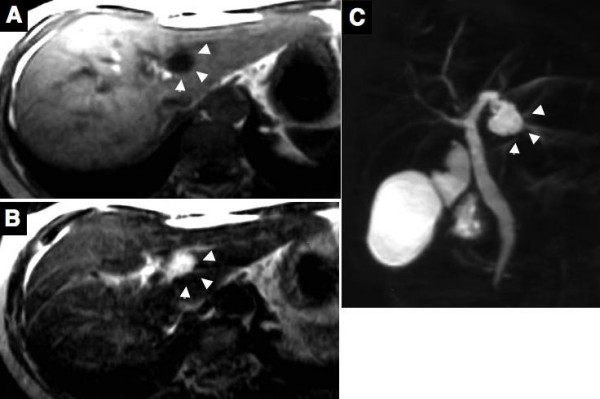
Magnetic resonance imaging (MRI) reveals the cystic lesion as low in the T1-weighted image (A) and as high in the T2-weighted image (B). MR cholangiography shows a cystic lesion at the left lobe of the liver, but a filling defect in the bile duct and a communication between the cystic lesion and bile duct could not be defined (C). Arrows head indicate the cystic lesion.
